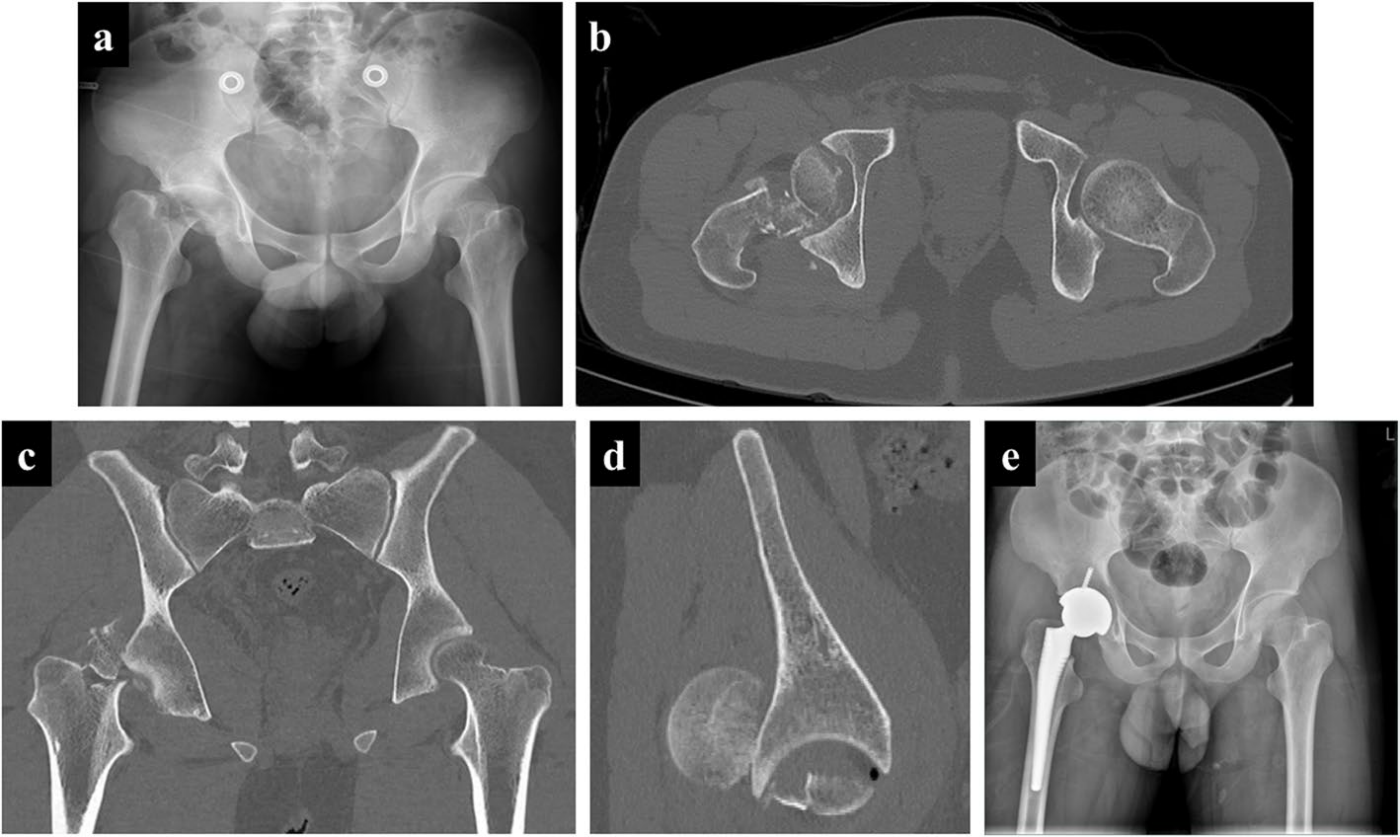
A representative case of the first subtype of iFHNF, namely posterior dislocation and fracture of the femoral head with simultaneous femoral neck fracture. A 24-year-old man involved in a severe traffic accident was diagnosed with femoral head and neck fractures based on X-ray (a) and CT images (b-d). A large extra-articular fragment of the femoral head and posterior hip dislocation can be observed (d). Total hip arthroplasty was performed (e)
